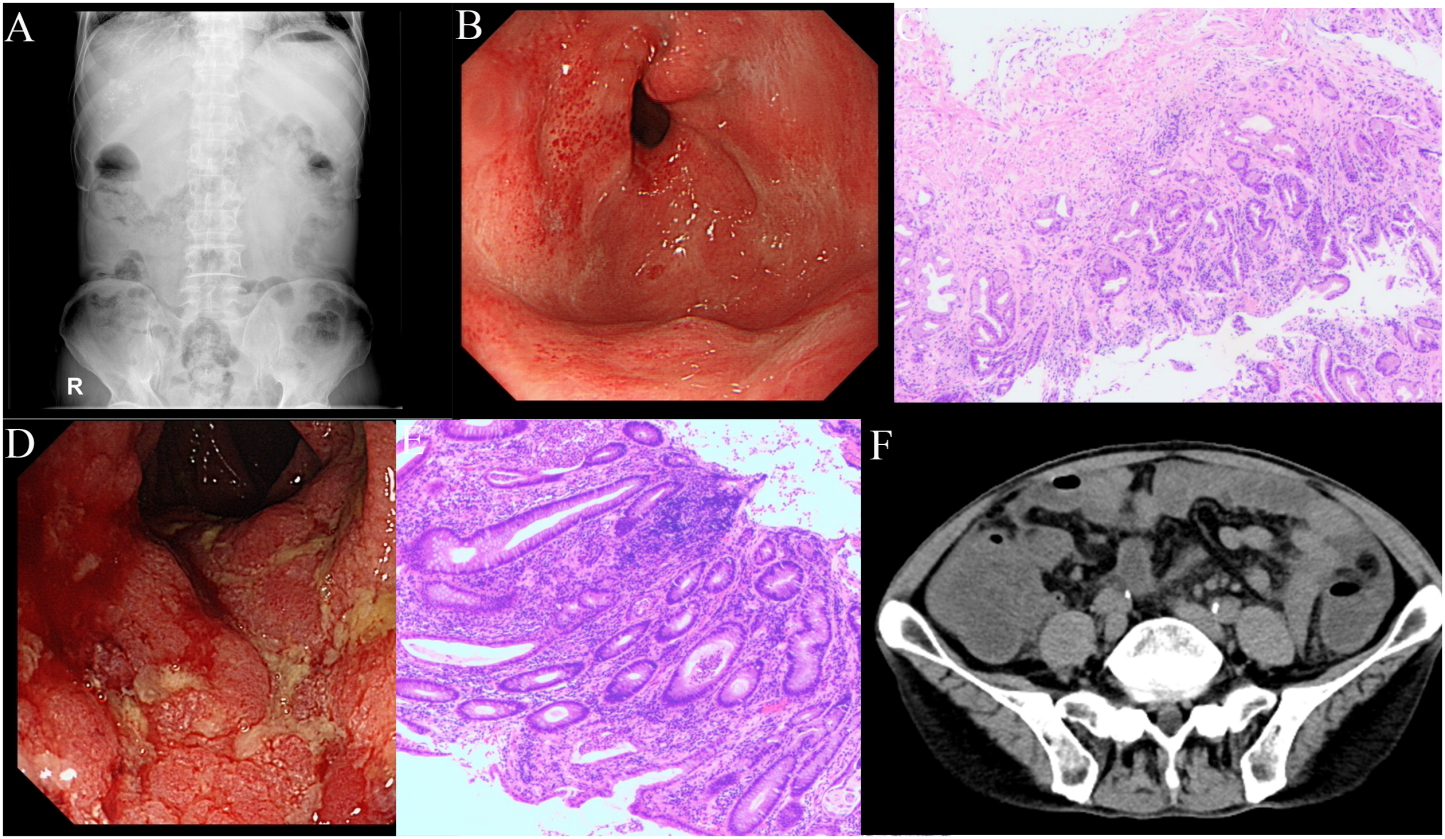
Clinical images during the treatment. (A) Plain abdominal x-ray shows no evidence of bowel distension or free intraperitoneal gas, but revealed increased colonic content and mild dilatation at the hepatic flexure of the right colon, along with gas-fluid levels. (B) Gastroscopy demonstrated features of chronic non-atrophic gastritis, with congested and edematous gastric folds, multiple ulcerative scars in the antrum, and flaky congestion in the duodenal papilla. (C) Pathological examination confirmed mild chronic mucosal inflammation with interstitial vascular congestion and a minor eosinophilic infiltrate. (D) Colonoscopy revealed congestion and edema of the colorectal mucosa, indistinct vascular patterns, friable mucosa prone to contact bleeding, and superficial erosions and ulcerations, leading to a diagnosis of colitis. (E) The pathology indicates a moderate level of chronic inflammation in the mucosa, with the presence of cryptitis and crypt abscesses. (F) Abdominal and pelvic scans revealed colonic dilation with fluid and gas accumulation.
